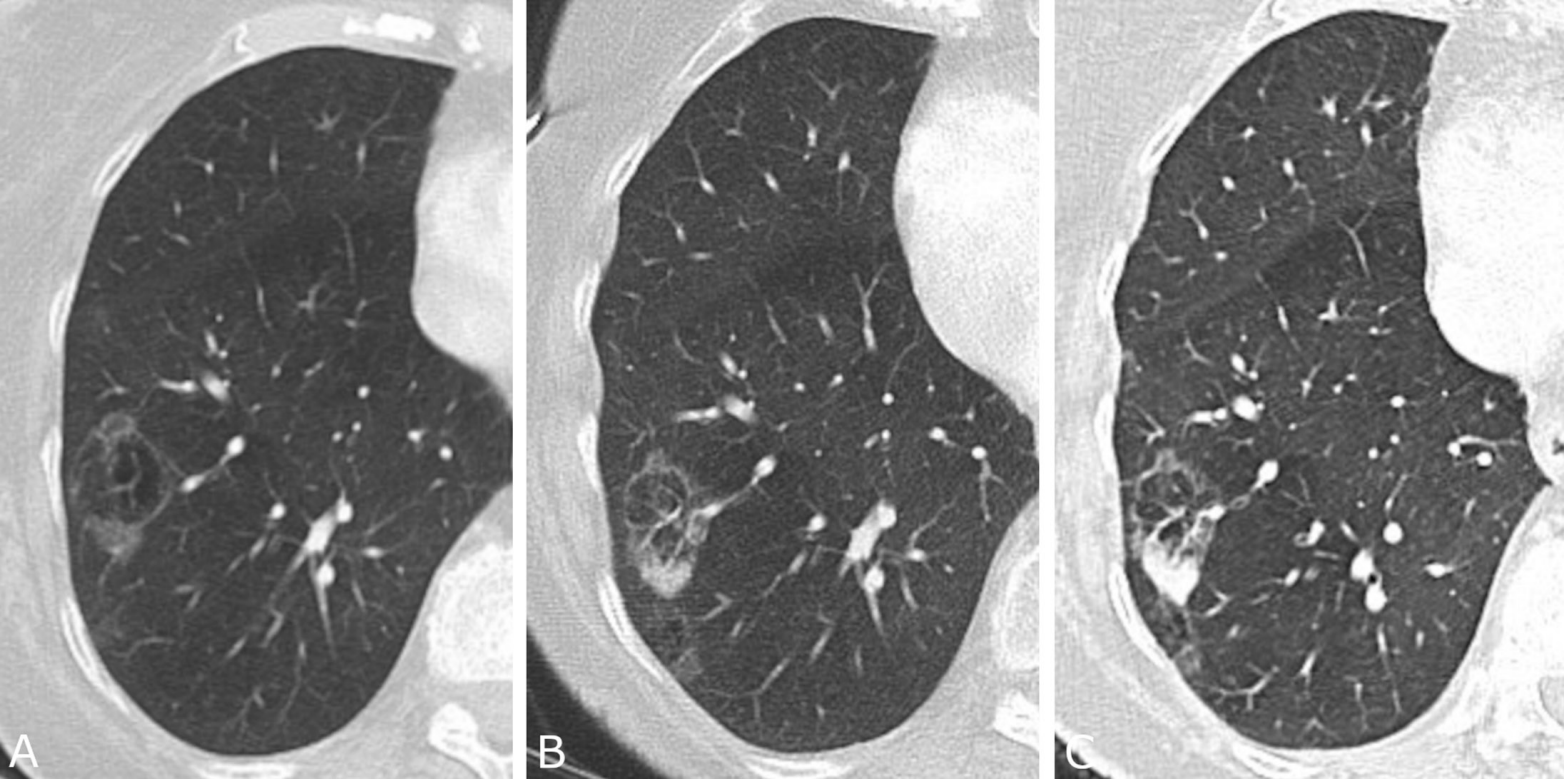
Progression of an atypical pulmonary cyst. Post‐contrast axial CT scans performed at baseline (A), 13 months (B) and 19 months (C) show progression of a peripheral opacity from ground glass to solid. There is no change in overall lesion size.
